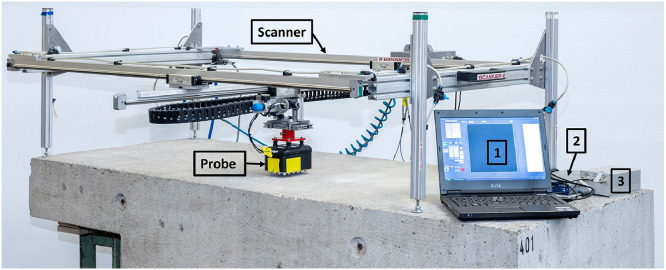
Automated scanner system and additional electronic measurement equipment used for the ultrasonic echo measurements (1: measuring laptop, 2: DAQ-Pad and 3: square wave pulser).
